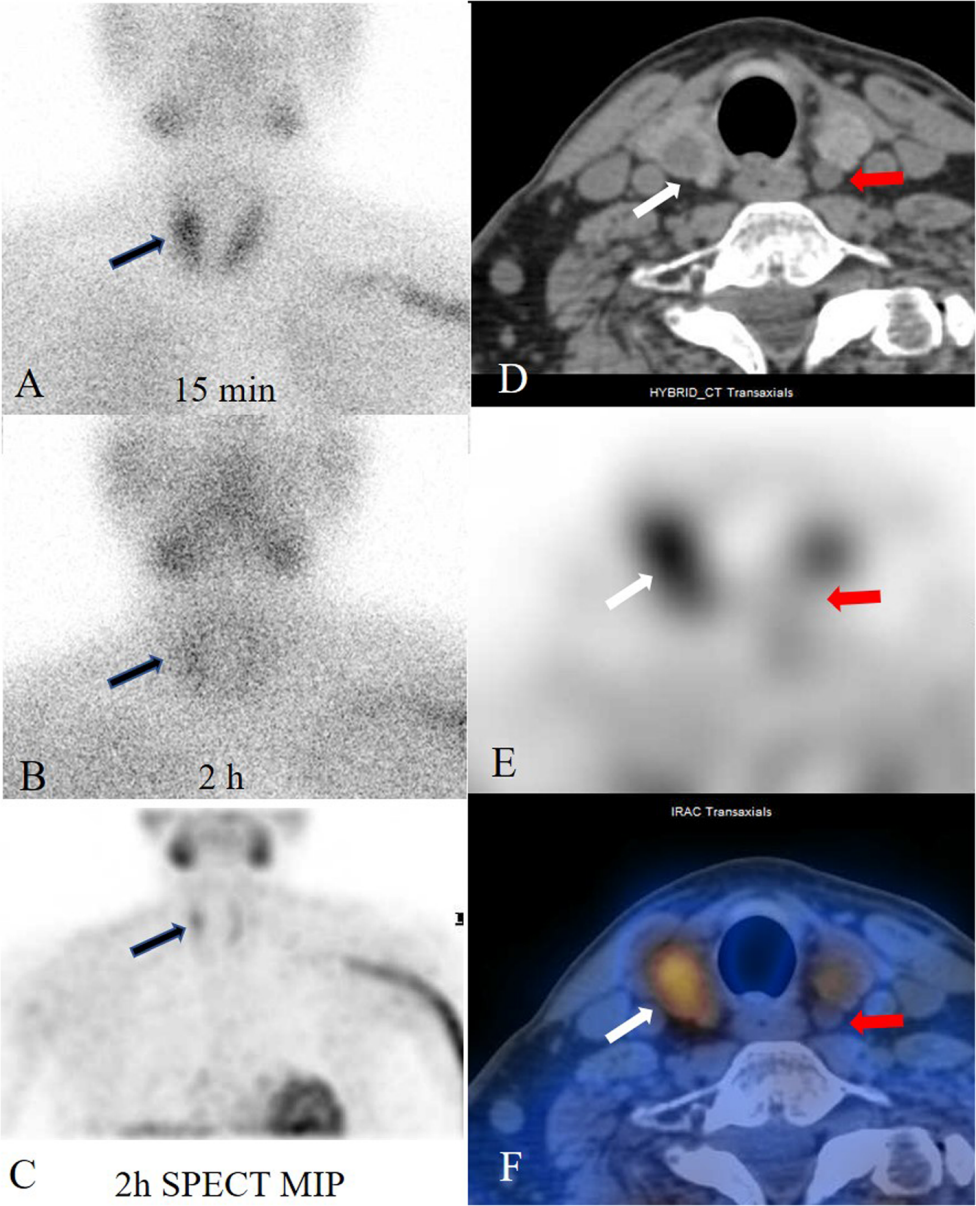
The ^99^mTcO4 MIBI dual-phase parathyroid scan detected elevated tracer uptake in the superior lobe of the thyroid in the early phase (a). A 2-h delayed image (b) showed mild retention of the tracer in the same region (black arrows). The SPECT/CT images at 2 h (c: MIP, maximum intensity projection; d: axial CT; e: SPECT; f: fused image) indicated that the focal tracer uptake was located in the right superior thyroid (white arrows). The lesion behind the superior left thyroid was a cystic nodule and had no radioactivity uptake (red arrows)
